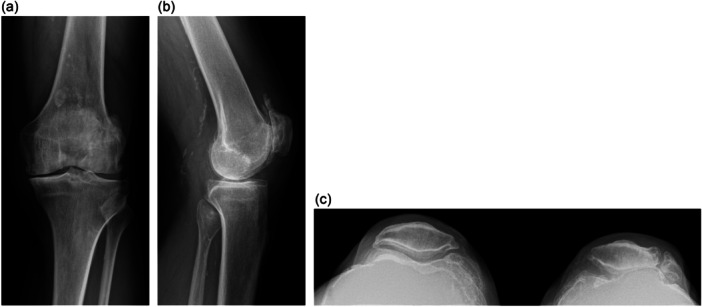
Clinical case: preoperative radiographs. (a) Weight‐bearing anteroposterior radiograph. (b) Lateral radiograph. (c) Axial patellofemoral (Merchant) views.
